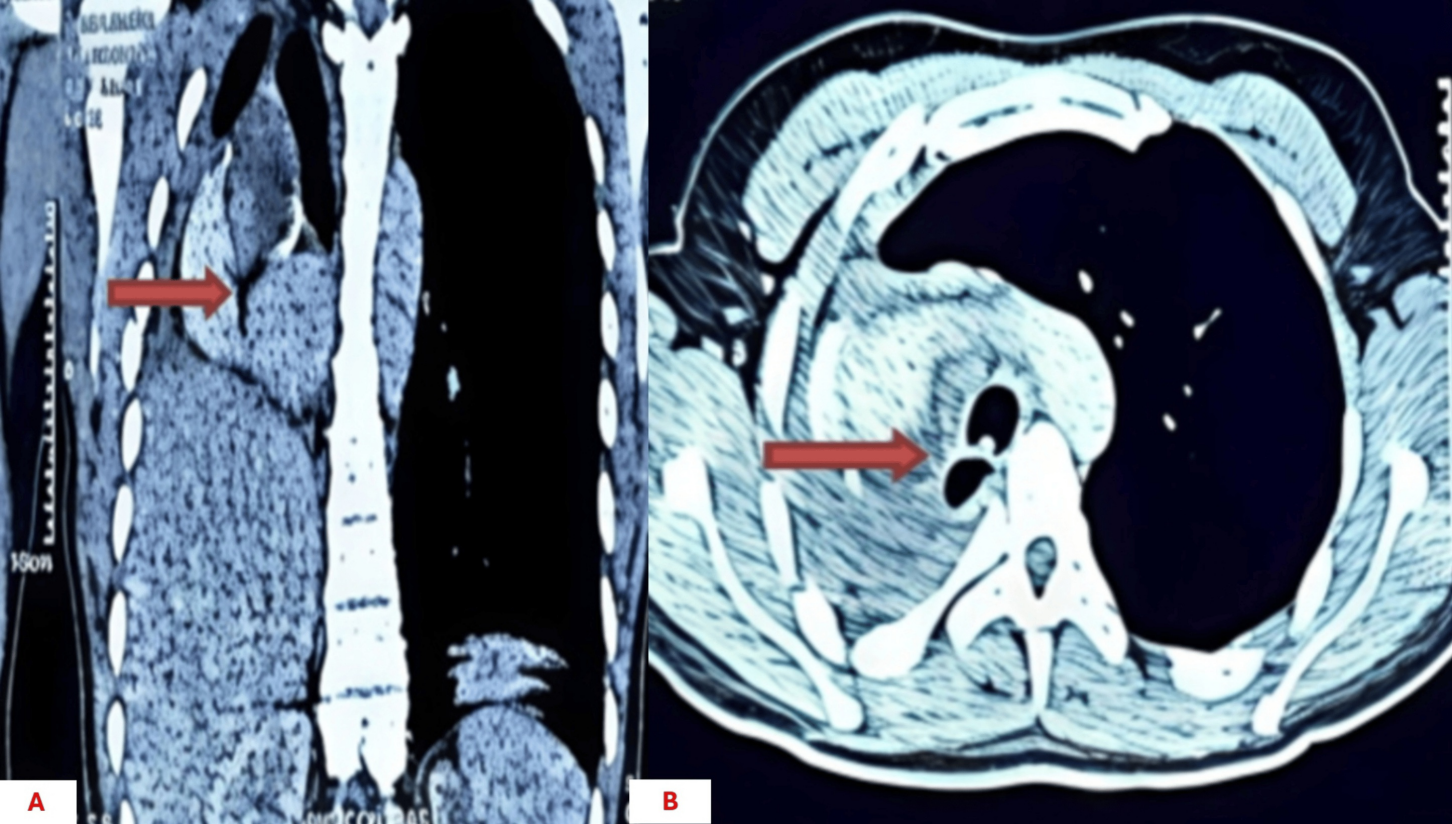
Postoperative thoracic CT scan. (A) Coronal CT image showing postoperative changes (red arrow) following right pneumonectomy. (B) Axial CT image demonstrating mediastinal shift and absence of residual tumor (red arrow).
